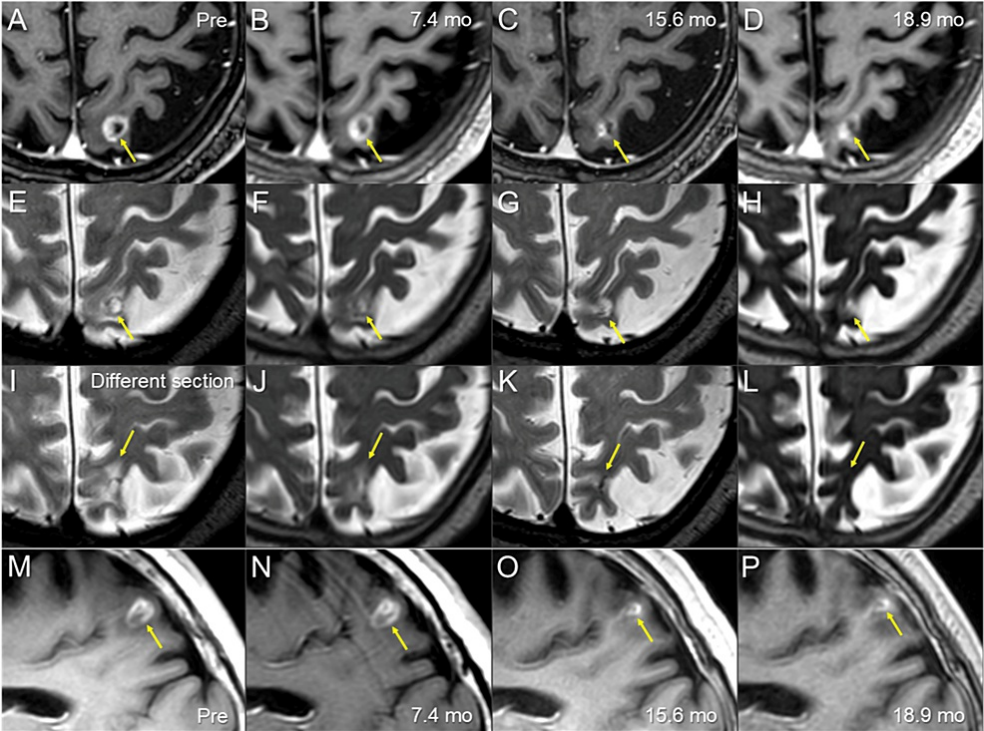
MRI findings before and after the re-radiosurgery for the local progression following prior radiosurgery The images show CE-T1-WIs (A-D, M-P); T2-WIs (E-L); axial images (A-L); sagittal images (M-P); before re-SRS (Pre) (A, E, I, M); at 7.4 months (mo) after the re-SRS initiation (B, F, J, N); at 15.6 months (C, G, K, O); and at 18.9 months (D, H, L, P) (A-P) These images are shown at the same magnification and coordinates (A-H, M-P) under co-registration and fusions; the section of the images I-L is different from that of the A-H. (A, E, I, M) The heterogeneously-enhancing lesion (arrows in A, M) and the corresponding ring-like lesion visible on the T2-WI (T2-mass, arrow in E), a T1/T2 match, are associated with perilesional edema (arrow in I). (B, F, J, N) At 7.4 months, the enhancing lesion (arrows in B, N) and the T2-mass remained almost unchanged; the surrounding high intensity became slightly less noticeable (arrow in J). (C, G, K, O) At 15.6 months, the enhancing effects and the lesion volume decreased significantly (arrows in C, O), the T2 mass became slightly obscured and some became low-intensity scar-like structures (arrows in G, K). The surrounding edema almost disappeared (arrows in G, K). (D, H, L, P) At 18.9 months, the enhancing effects became slightly more noticeable (arrows in D, P); the T2 mass became more obscure and the surrounding edema continued to disappear (arrows in H, L) MRI: magnetic resonance imaging; CE: contrast-enhanced; WIs: weighted images; SRS: stereotactic radiosurgery; T2 mass: a visible mass on T2-WIs
